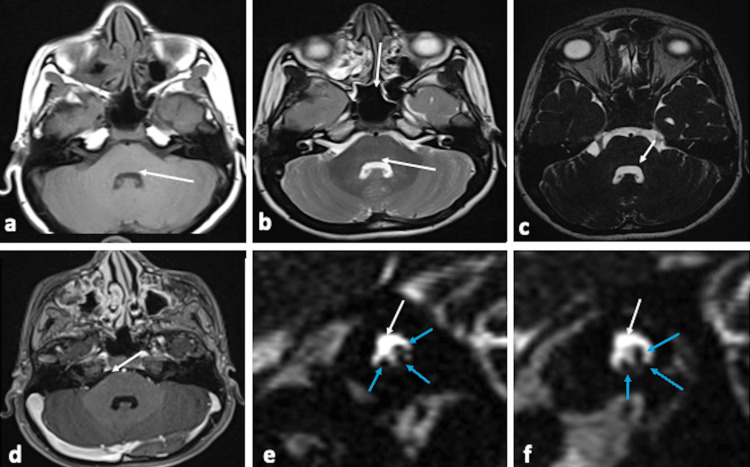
Magnetic resonance imaging (MRI) images showed the imaging features of Moebius syndrome. MRI Axial T1 (a), T2 (b), and FIESTA (fast imaging employing steady-state acquisition) (c) images showed flattening of the floor of the fourth ventricle with the absence of bilateral facial colliculus (white arrows), and post-contrast T1 (d) image showed absent cisternal segments of bilateral abducens nerves (white arrow). Sagittal MRI image (FIESTA sequences) (e,f) showed the absence of the facial nerves (white arrows in (e,f)) with intact superior, inferior vestibular, and cochlear nerves (blue arrows in (e,f)) within both internal auditory canals.
